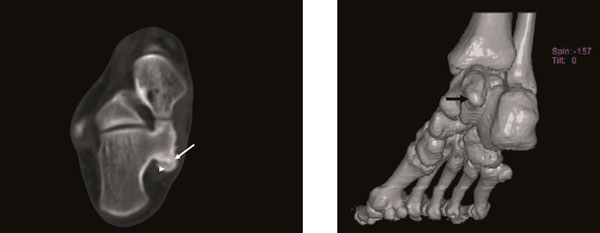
Axial CT scan (A) and three-dimensional (3D) imaging (B) taken at the 6-month the first-operative of the foot shows increase in the lesion sizes (18 × 12 mm). (arrow: osteochondroma, arrowhead: cartilage cap).
